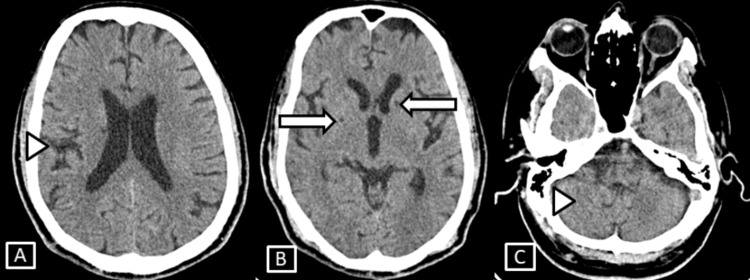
Case 3 CT brain axial images. Images show mild age-related neuroparenchymal atrophic changes involving the sulcal, extra-axial CSF spaces and along the cerebellar foliae (A,C) represented by the white arrowheads. A few chronic lacunar infarcts seen involving the right lentiform nucleus and left external capsule (B) represented by the white arrows. CSF: cerebrospinal fluid
